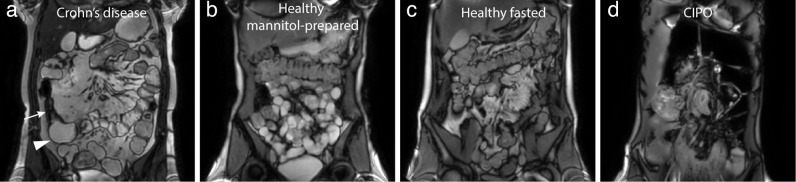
MRI examples of the included populations with different small bowel characteristics. (a) shows a Crohn’s disease patient with a stricture (tailed arrow) and prestricture dilation (arrowhead), (b) a mannitol-prepared healthy subject, (c) a healthy fasted subject and D) a CIPO patient with dilated small bowel loops.
